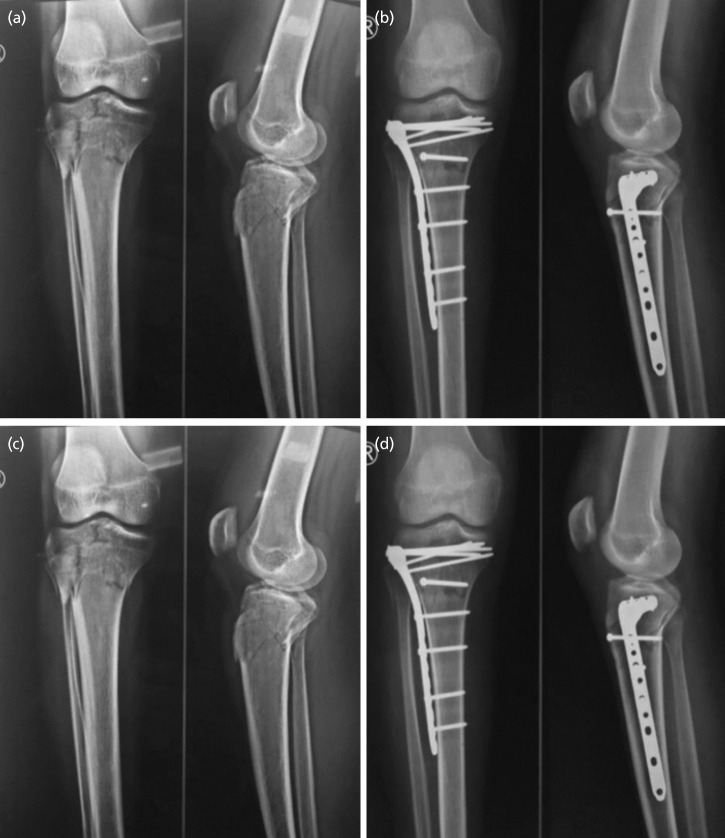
Follow-up series of radiographs. (a) Pre-operative. (b) Immediate post-operative. (c) 2 months post-operative. (d) 6 months post-operative.
